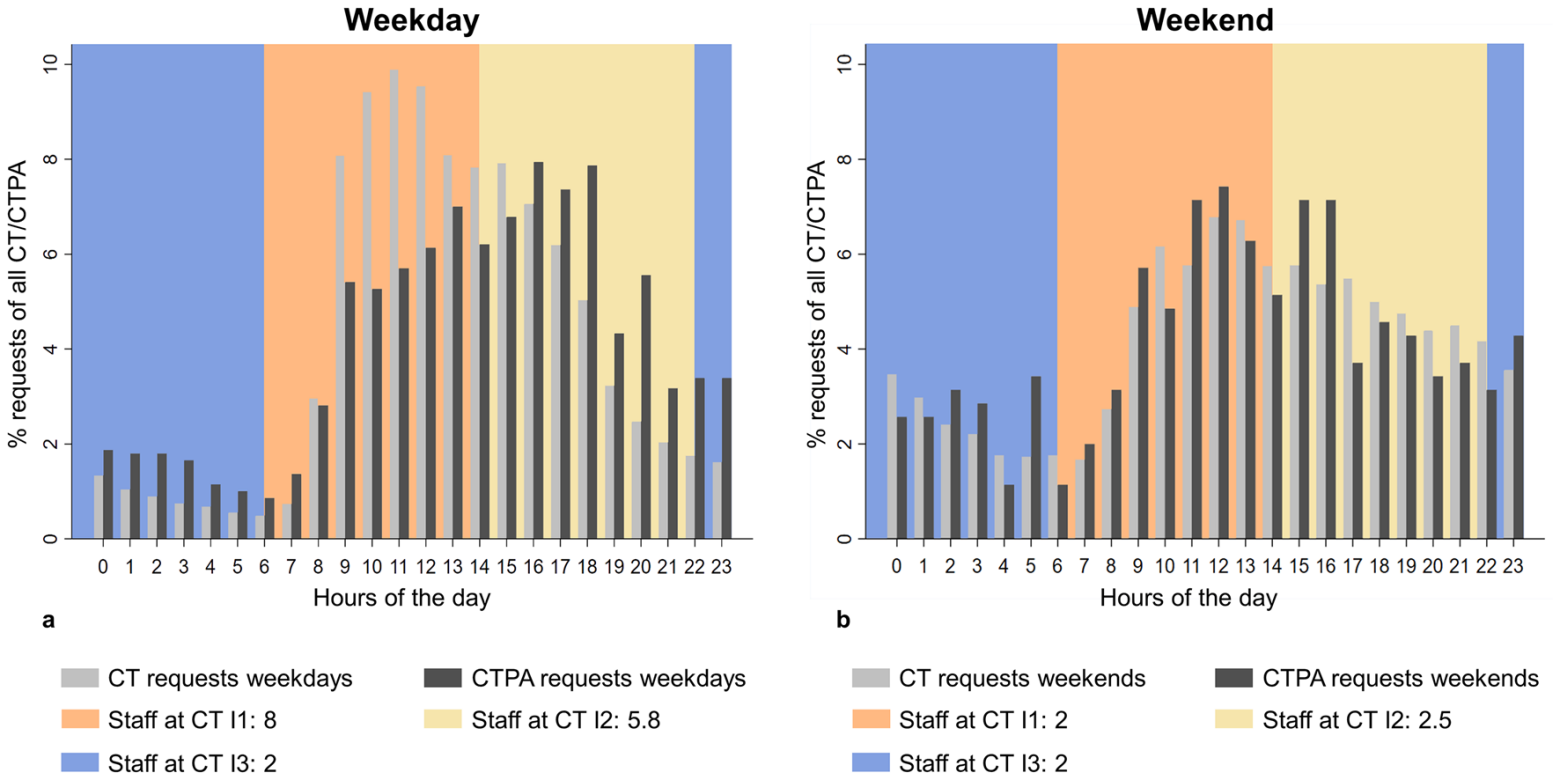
Relative demand for CT/CTPA per hour of the day during the study period and median number of staff available during intervals I1 (6 am–2 pm), I2 (2–10 pm), and I3 (10 pm–6 am) on weekdays (a) and weekends (b).
